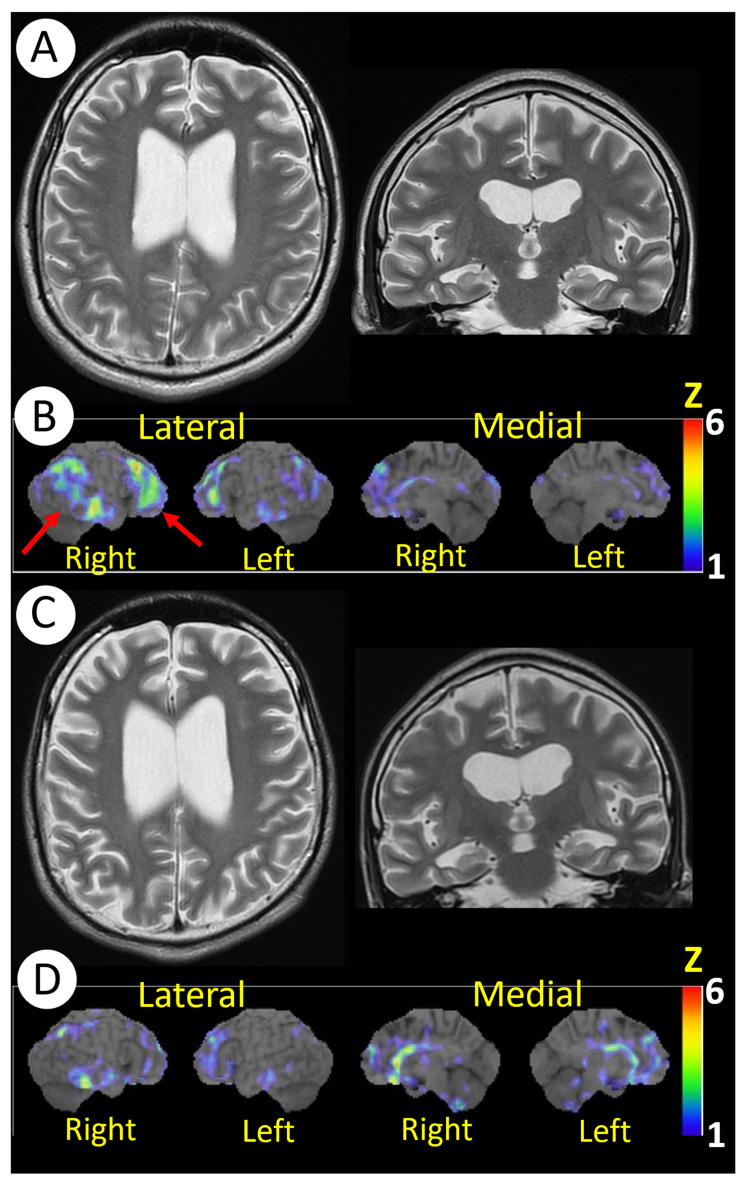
MRI and SPECT The findings of brain magnetic resonance imaging (MRI) and cerebral blood flow single-photon emission computed tomography using N-isopropyl-p-[^123^I] iodoamphetamine (SPECT). Twenty-seven (27) months after the onset: (A) MRI shows atrophy of the bilateral frontal lobes and mesial temporal lobes and dilatation of the lateral ventricles. (B) SPECT reveals hypoperfusion in the right-dominant frontal and parieto-temporal cortices (arrows). Thirty-six (36) months after the onset and nine months after the ketogenic diet initiation: (C) MRI shows no changes compared to (A) before the introduction of the ketogenic diet. (D) SPECT shows the improvement of hypoperfusion in the frontal and parieto-temporal cortices. The Z-score on SPECT is higher as the degree of decrease in cerebral blood flow is larger than that of an age-matched normal database.
